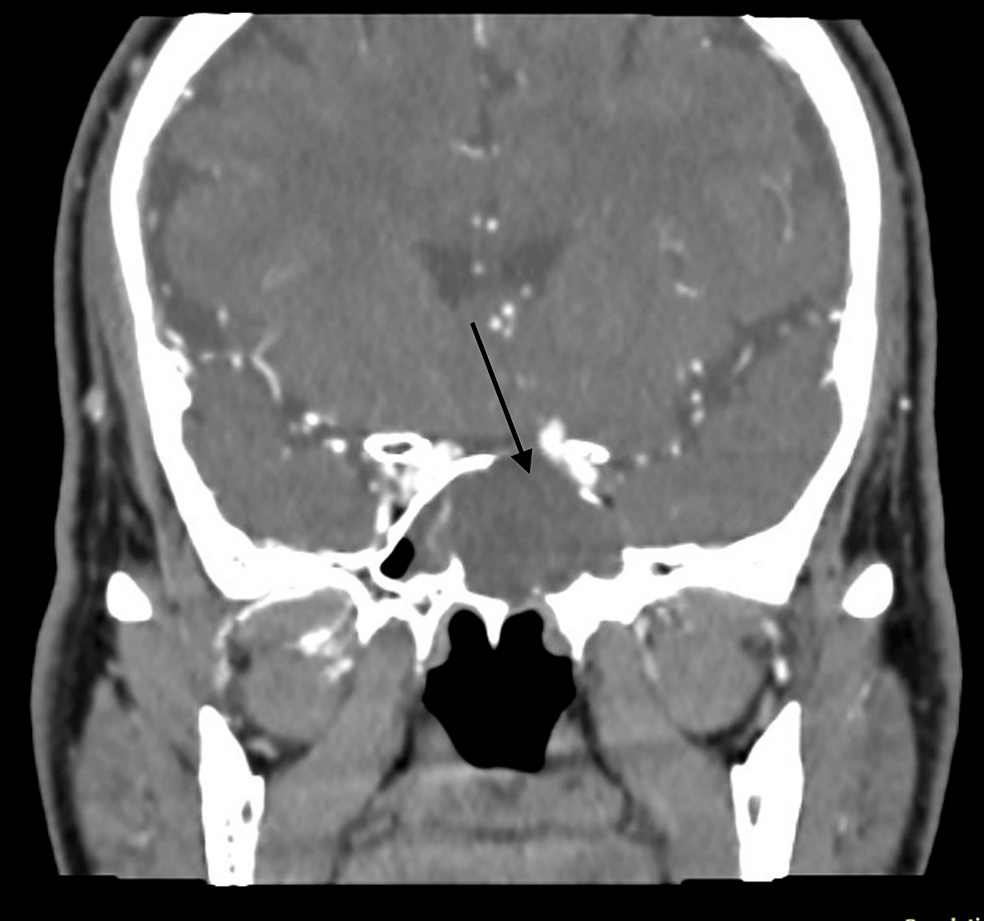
CT scan of the paranasal sinuses in the coronal plane The image shows the same lesion with an erosion of the lateral and superior wall of the sphenoid sinus on the left side, no intracranial involvement can be appreciated (black arrow).
